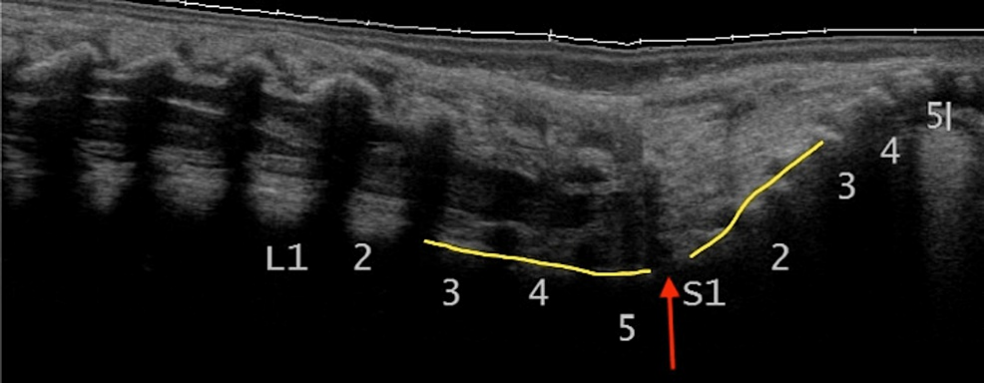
Lumbosacral junction Longitudinal ultrasound image demonstrating the lumbosacral junction (red arrow) as an abrupt change in angulation between L5 and S1 Radiological image obtained by the authors
